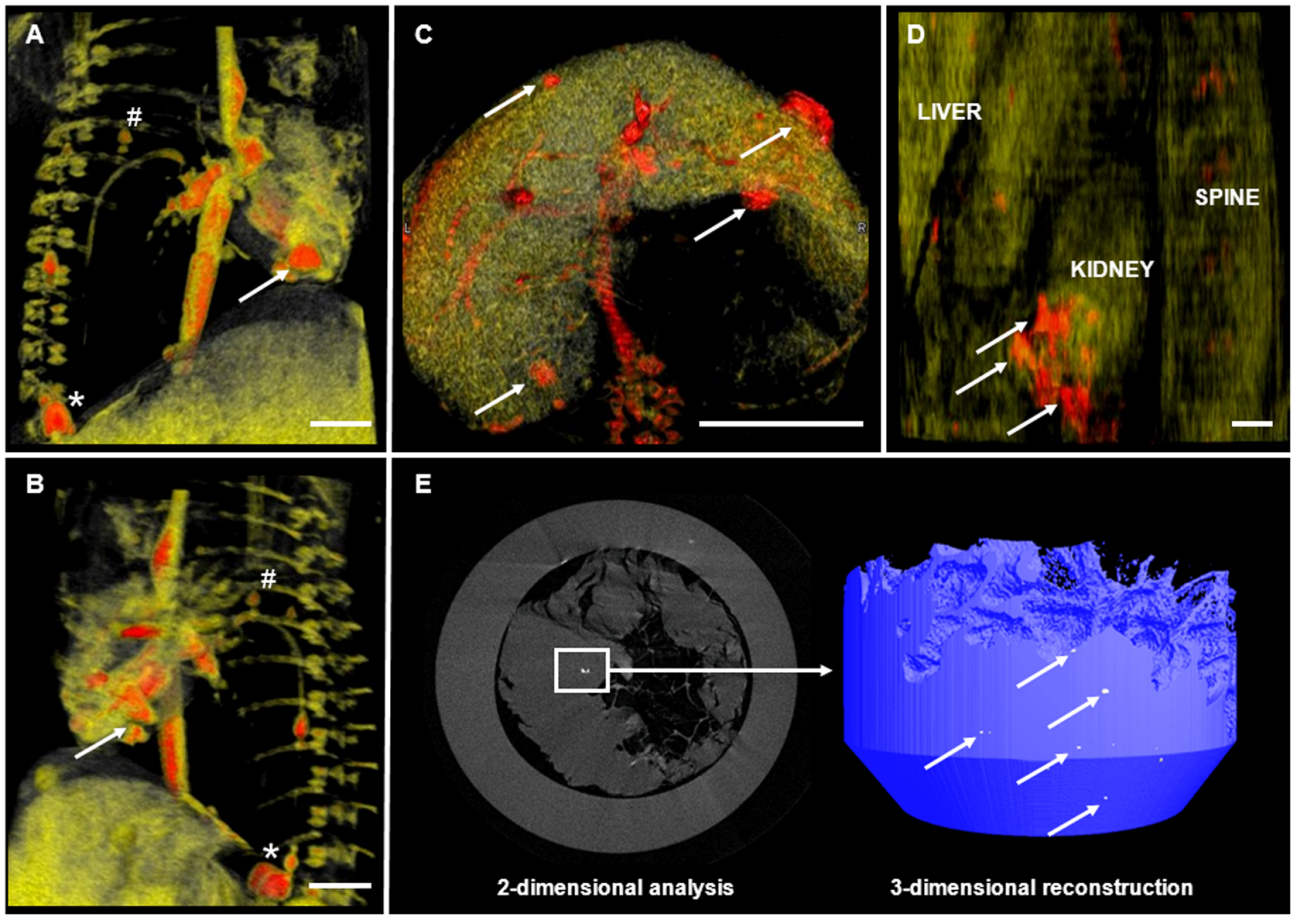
Tracking of CM-DiI^+^/MPIO^+^ human ATMSCs and BMMSCs on three-dimensional reconstruction MRI and Micro CT Scanning. Exemplary three-dimensional reconstruction analysis confirmed labeled cell clusters of human CM-DiI^+^/MPIO^+^ ATMSCs after intramyocardial transplantation in the anterior-lateral and septal ventricular wall (A and B; *white arrows; anterior-lateral and septal view*). In addition, labeled cells were also detectable in the costal/para-vertebral area (A and B; *white ) and in the Sinus phrenico-costalis (A and B; *white #*) indicative that the cells had been distributed in the fetal thorax. Abdominal 3D reconstruction analysis showed labeled cell clusters of CM-DiI^+^/MPIO^+^ human BMMSCs following intra-peritoneal injection within and around the liver (C; *white arrows; anterior-inferior view*) as well as in the anterior the Gerota's fascia (D; *white arrows; lateral view*). The intra-myocardial presence of CM-DiI^+^/MPIO^+^ human ATMSCs could be further confirmed showing several labeled cell clusters in the fetal myocardium on 2-dimensional as well as 3-dimensional high-resolution Micro CT analysis (E). *Scale Bar: 2 cm*.
